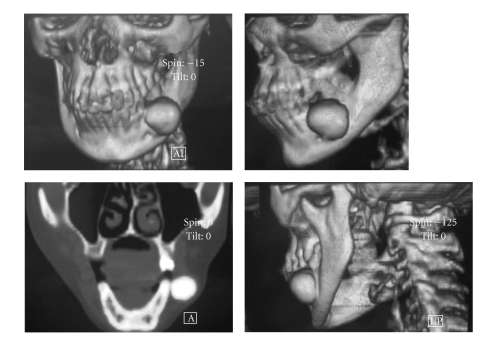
Coronal Computed tomography (CT) showing a large, well-circumscribed, pedunculated mass attached to the buccal surface of the left mandibular body. Three-dimensional reconstruction image showing localisation and extending of the lesion.
